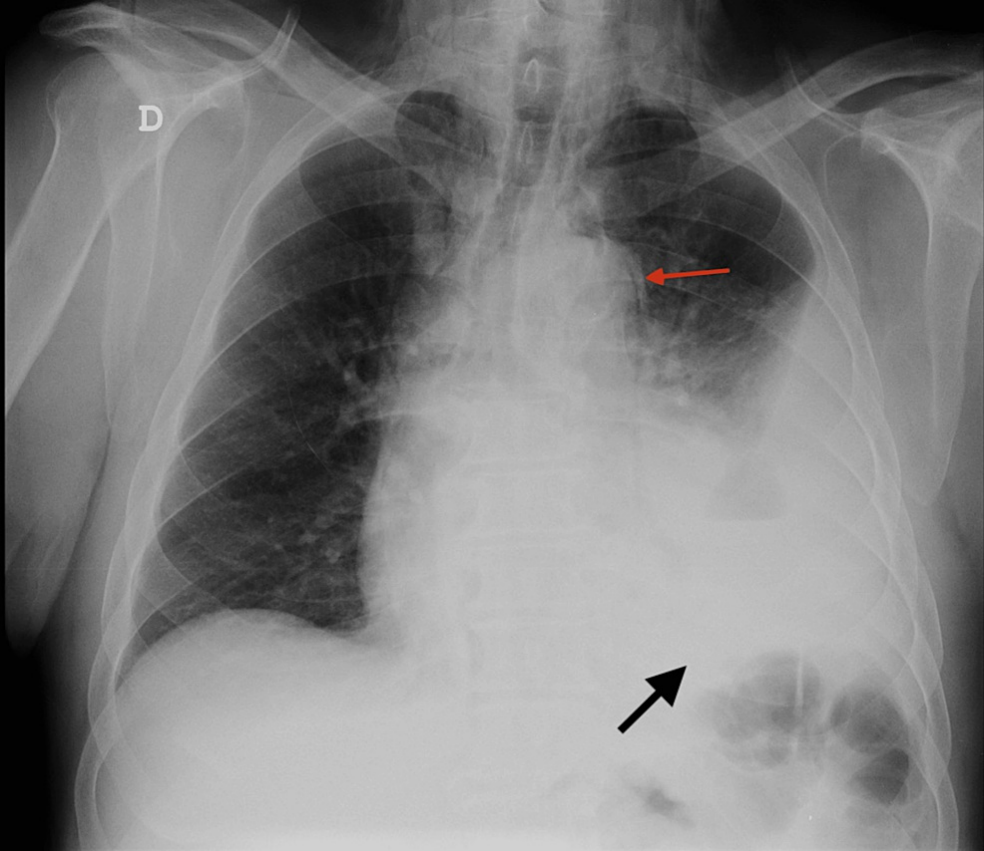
Chest X-ray revealing a pneumomediastinum (red arrow) and a left pleural effusion (black arrow).
